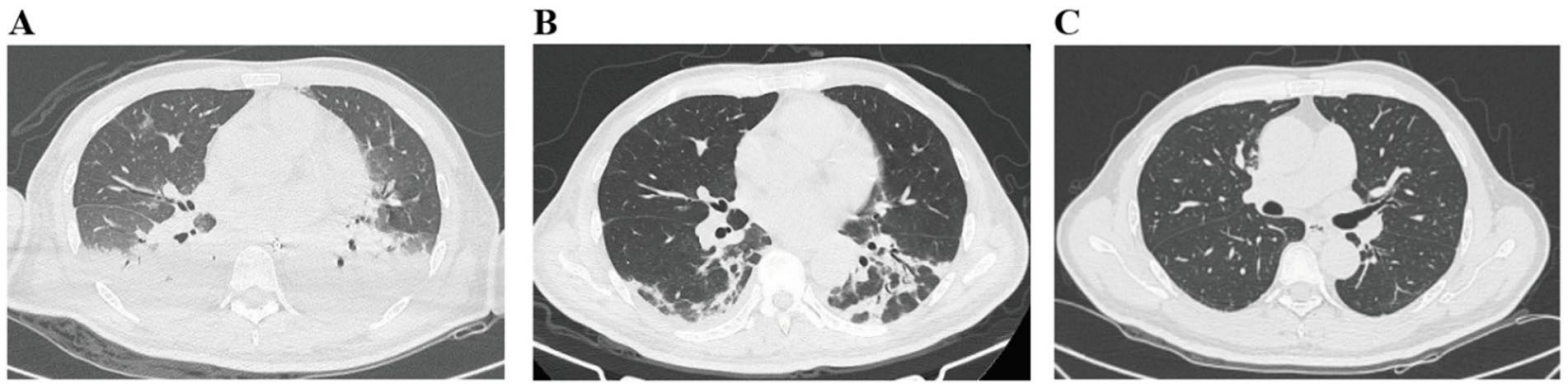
Improvement in lung imaging before and after standardized treatment, as shown on chest CT. Panel A shows a chest CT scan with patchy areas of haziness in both lungs. Panel B displays a chest CT scan with multifocal consolidations and ground-glass opacities, especially in the lower lung zones. Panel C depicts a chest CT scan of clear lungs with normal parenchymal markings and no significant opacities.
